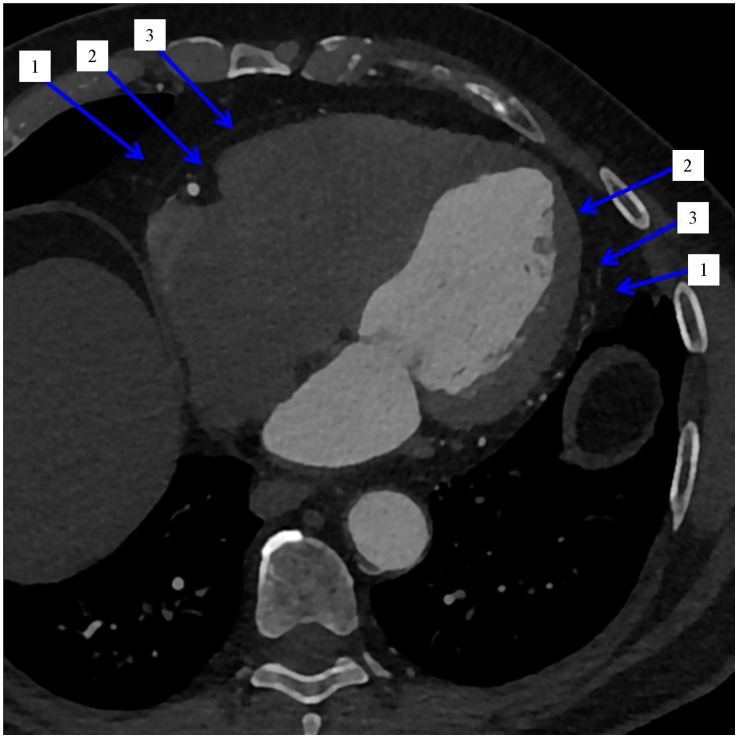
Cardiac computed tomography. Axial reconstruction. Blue arrows indicate: 1. Pericardial adipose tissue. 2. Epicardial adipose tissue. 3. Pericardium.
